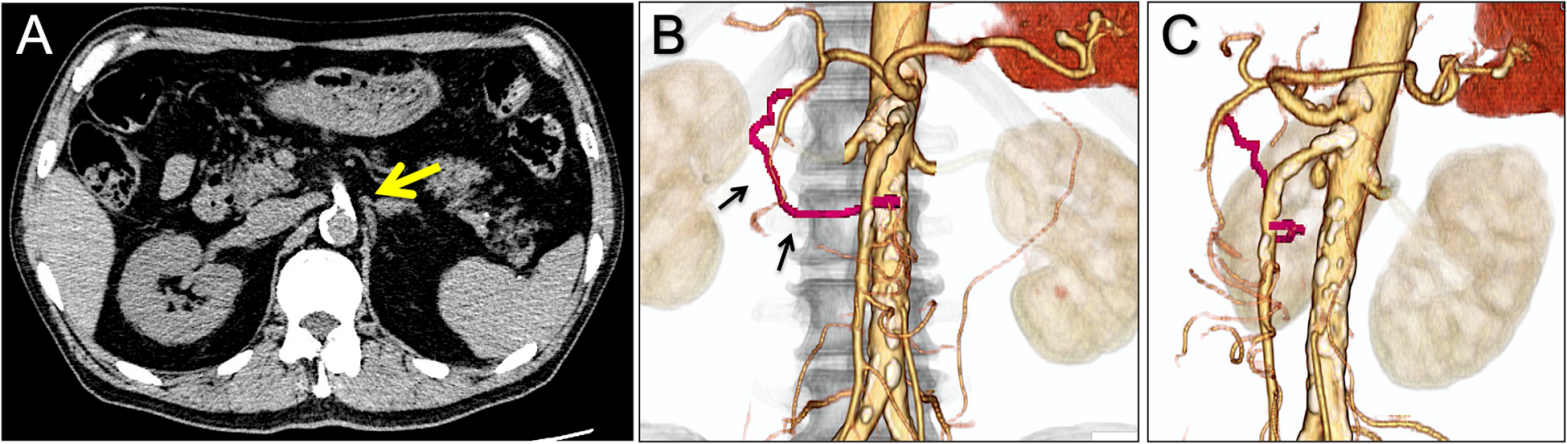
Computed tomography. A, Noncontrast computed tomography (CT) revealed a heavily calcified occlusion in the ostium of the superior mesenteric artery (a yellow arrow). B and C, Contrast-enhanced CT showed a pancreaticoduodenal arcade in the anteroposterior view (black arrows) and in the angulated 65-degree left anterior oblique position
